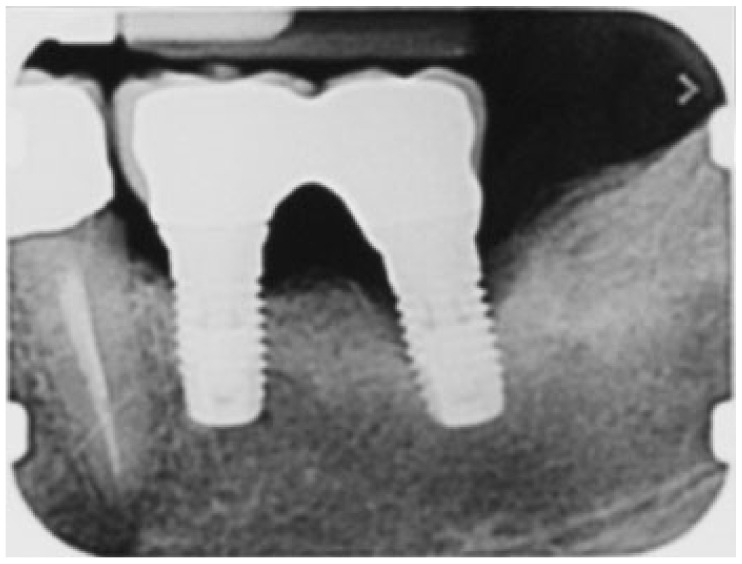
Rx periapical before peri-implant therapy: infra-bony defect around the distal implant.
